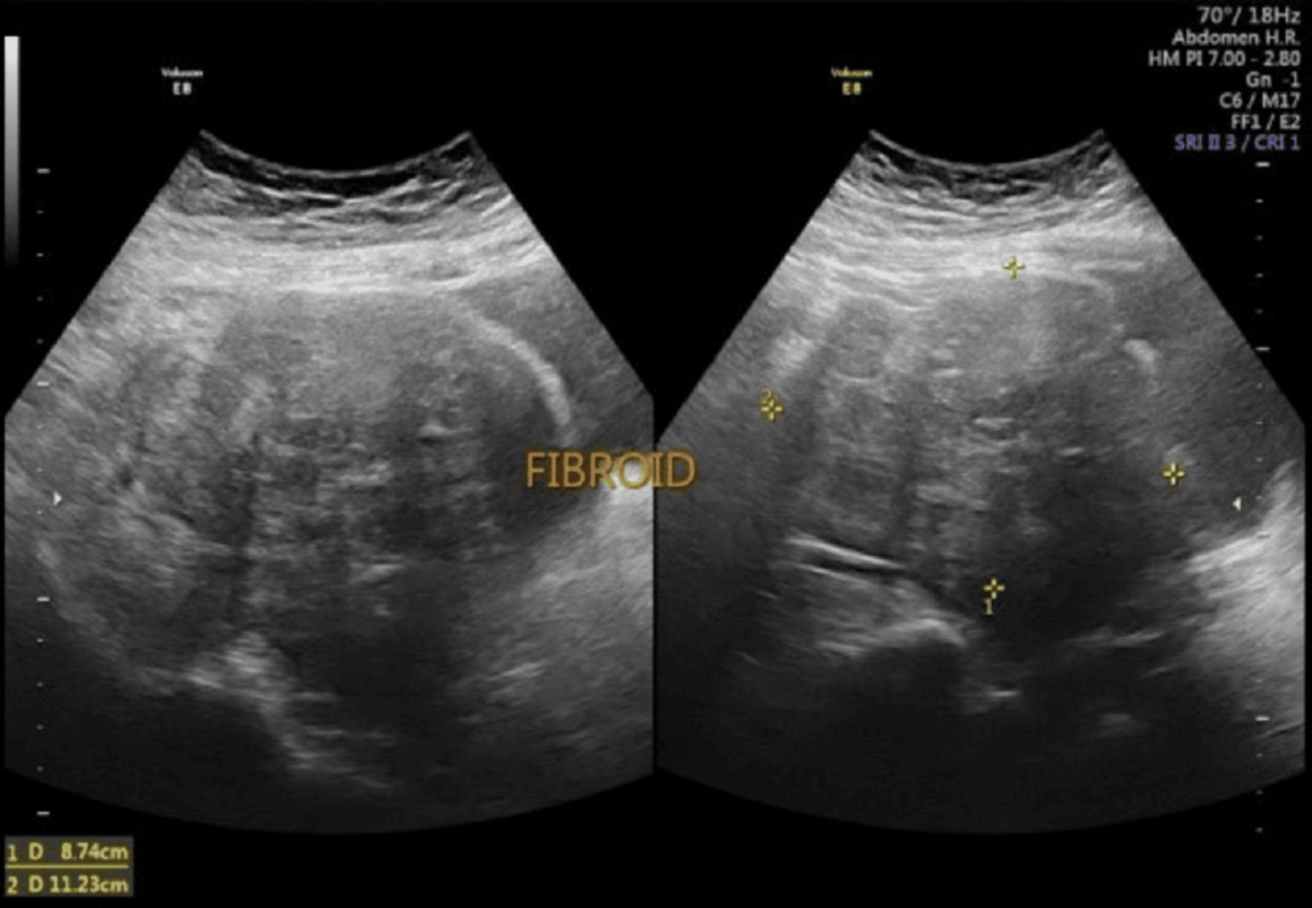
Ultrasound of 11.7 cm lower segment fibroid at 11 weeks of pregnancy
